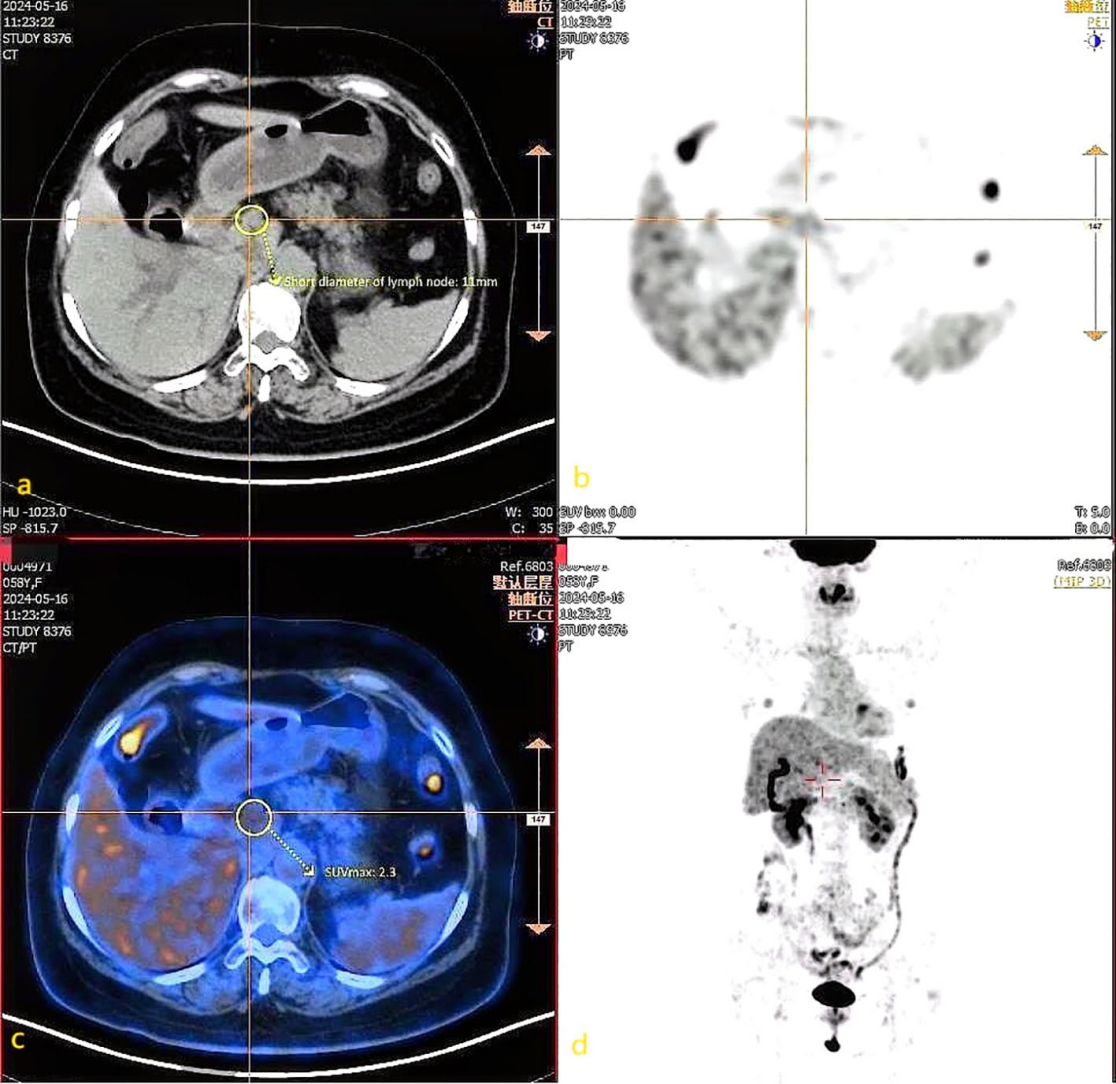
CT images and ^18^F-FDG PET-CT images of metastatic lymph nodes in liver cancer patients. (a) CT axial image shows a lymph node with a short-axis diameter of 11 mm. (b) PET images primarily reflect the metabolic activity of tissues, and the labeled areas may indicate regions with potentially abnormally increased metabolism. (c) The PET-CT fusion image shows the marked lymph node with an SUVmax of 2.3. (d) PET whole-body maximum intensity projection images can comprehensively display the metabolic activity of the entire body.
